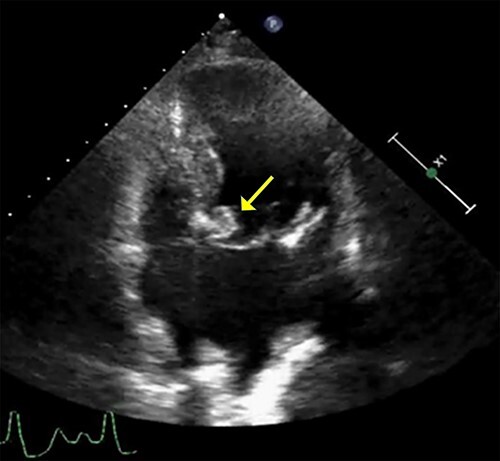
Transthoracic echocardiogram. A well-defined densely calcified mass noted on the anterior mitral leaflet in the apical four-chamber view.
